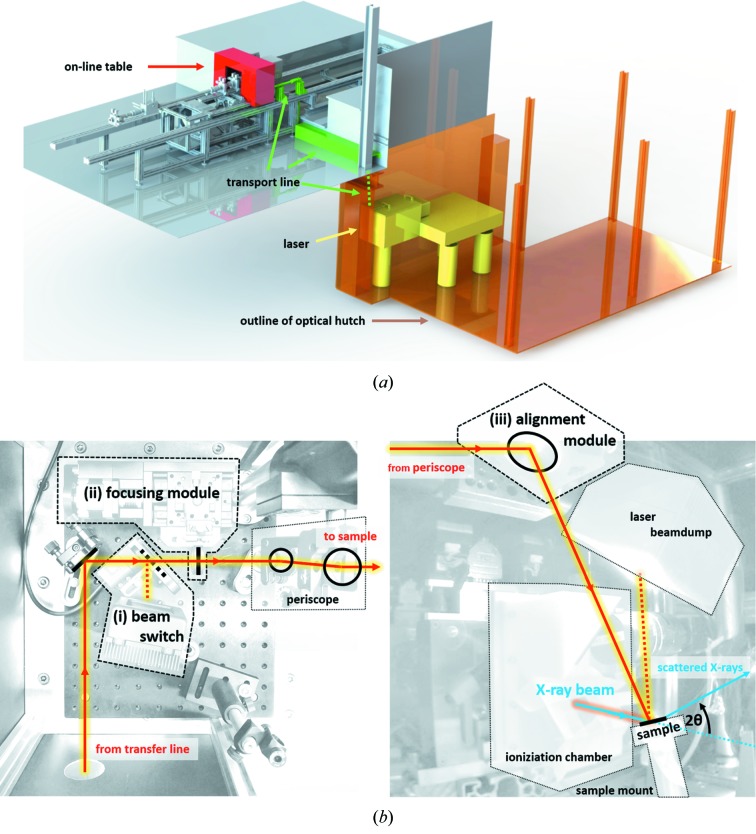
(*a*) Sketch of the laser infrastructure implemented at the Austrian SAXS beamline for pump–probe experiments. (*b*) Photographs of optical elements inside the on-line table enclosure in grazing-incidence configuration (left: top view; right: side view). Here, the laser trajectory is highlighted in red while the X-ray beam is shown in blue.
